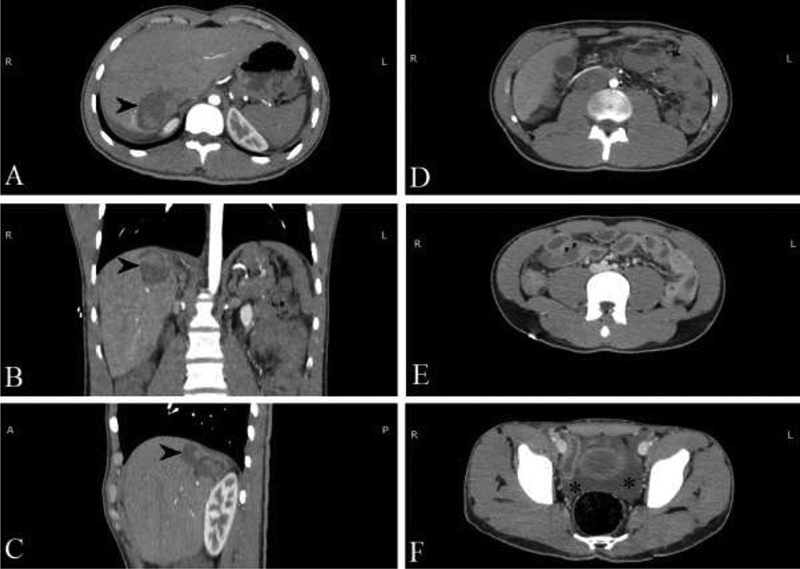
Computed tomography (CT) scan of the abdomen and pelvis + intravenous (IV) contrast. (
